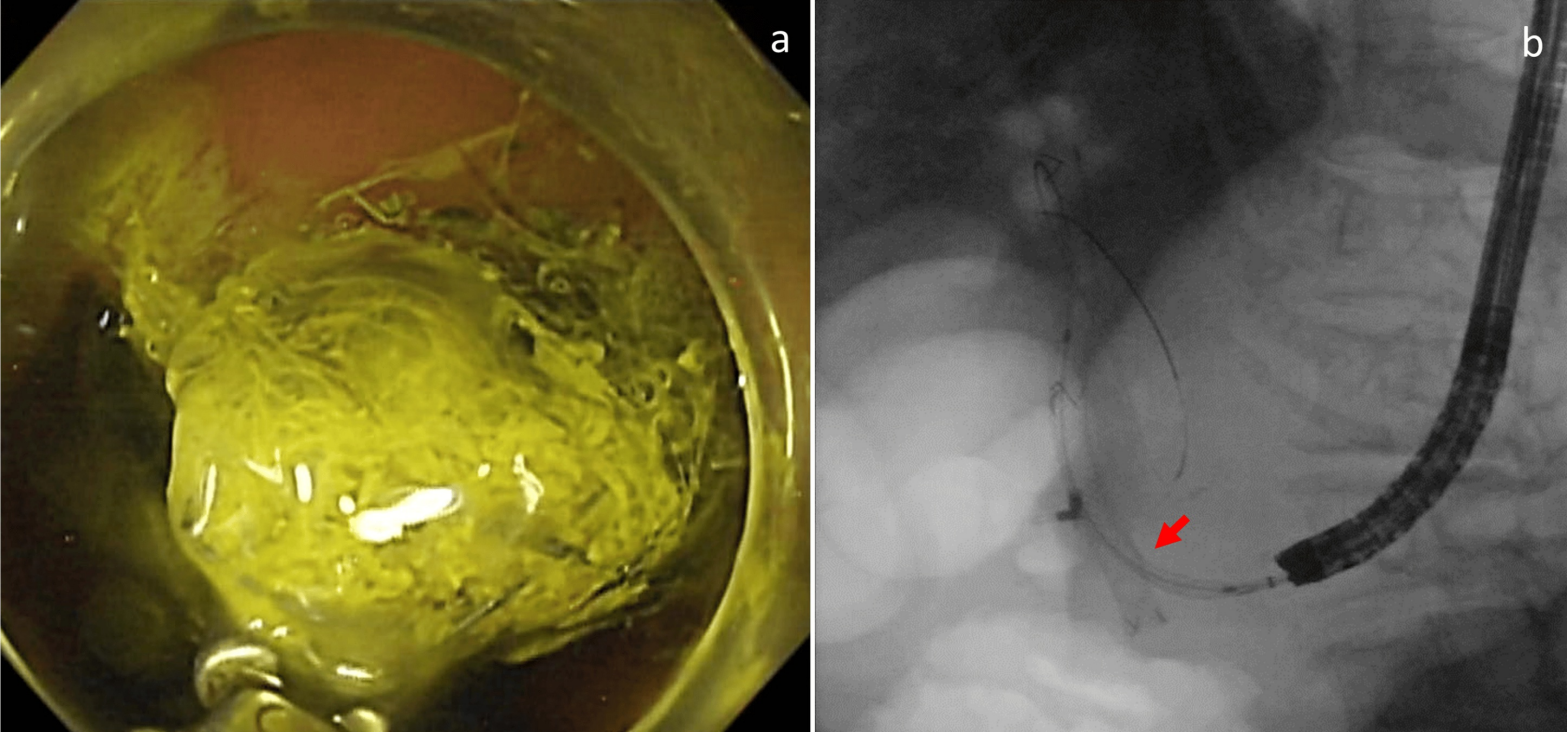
Images at the time of re-intervention a: endoscopy performed three days after endoscopic ultrasound-guided hepaticogastrostomy shows a short stent in the stomach that is on the verge of abdominal cavity migration; b: fluoroscopy shows the insertion of an additional metal stent through the mesh of the existing stent (red arrow).
